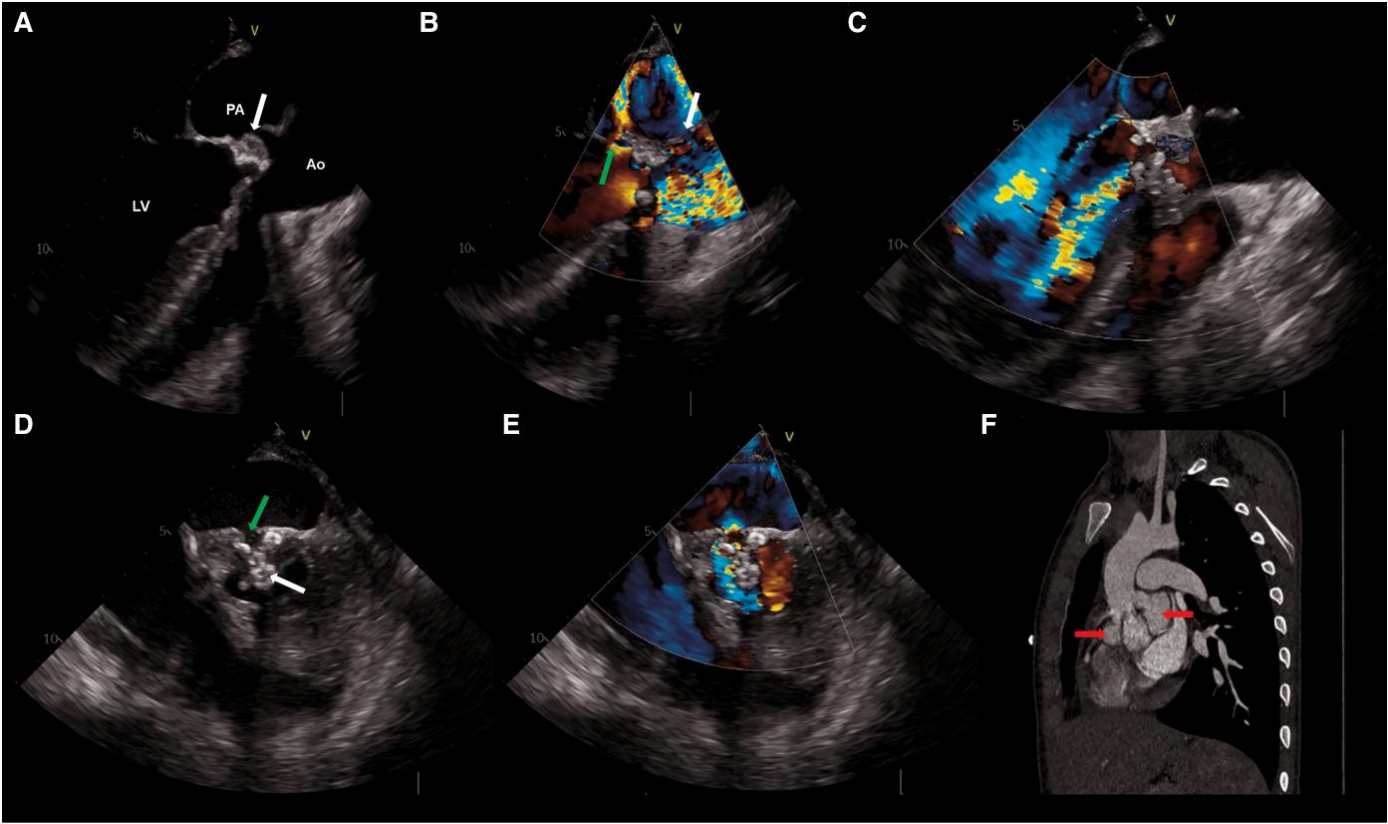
Transoesophageal echocardiogram and thoracic computed tomography scan. (*A*) Mid-oesophageal long-axis transoesophageal echocardiogram view showing large vegetations (white arrow) on the aortic valve leaflets and an associated sinus of Valsalva pseudoaneurysm. (*B*) Colour Doppler in the same transoesophageal echocardiogram view demonstrating turbulent aortic valve flow and the pseudoaneurysm (white arrow) draining into the left ventricle (green arrow). (*C*) Colour Doppler in the same transoesophageal echocardiogram view revealing severe aortic insufficiency during diastole. (*D*) Mid-oesophageal short-axis transoesophageal echocardiogram view highlighting vegetations (white arrow) on a bicuspid aortic valve and a large sinus of Valsalva pseudoaneurysm (green arrow). *(E)* Colour Doppler in the short-axis transoesophageal echocardiogram view showing aortic regurgitation and flow directed into the pseudoaneurysm. (*F*) Reconstructed sagittal computed tomography image of the aortic root demonstrating significant distortion and the presence of two pseudoaneurysms (red arrows), collectively forming the ‘garlic sign’. Label: Ao, ascending aorta; LV, left ventricle; PA, pseudoaneurysm.
